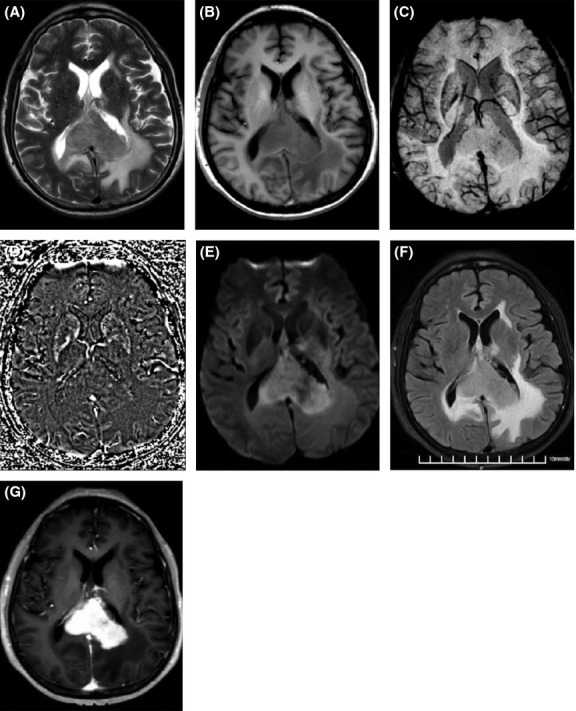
A 62-year-old male with diffuse large B cell lymphoma. One lesion was located in splenium on T2WI (A) and T1WI (B). There were no vessels and microhemorrhage shown on T1WI and T2WI. However, SWI-MinIP (C) showed microhemorrhage with sharp border in the lesion and high signal on phase image. (D) The lesion showed hyperintense signal both on DWI (E) and FLAIR (F), and demonstrated solid, intense, and homogeneous enhancement on contrast-enhanced T1-weighted image (G).
